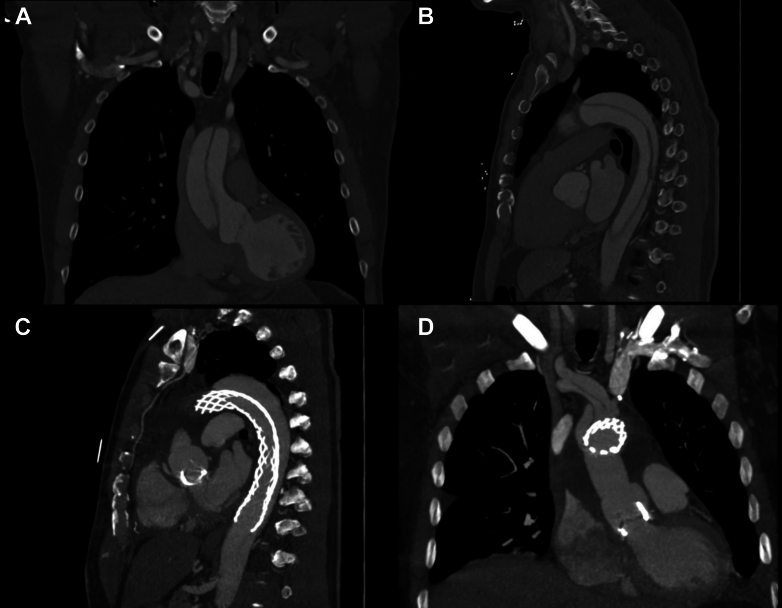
Preoperative Computed Tomography Scans Preoperative computed tomography (CT) demonstrating an acute type A aortic dissection (A and B). Postoperative CT following ascending aortic repair and AMDS Hybrid Prosthesis implantation (C and D).
